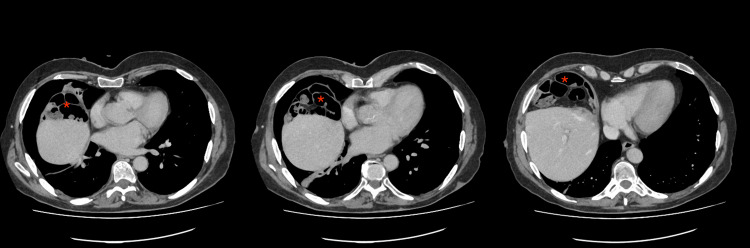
Axial CT showing the Chilaiditi sign: interposition of the colon between liver and diaphragm. The asterisk (*) marks the bowel segment situated above the liver.
